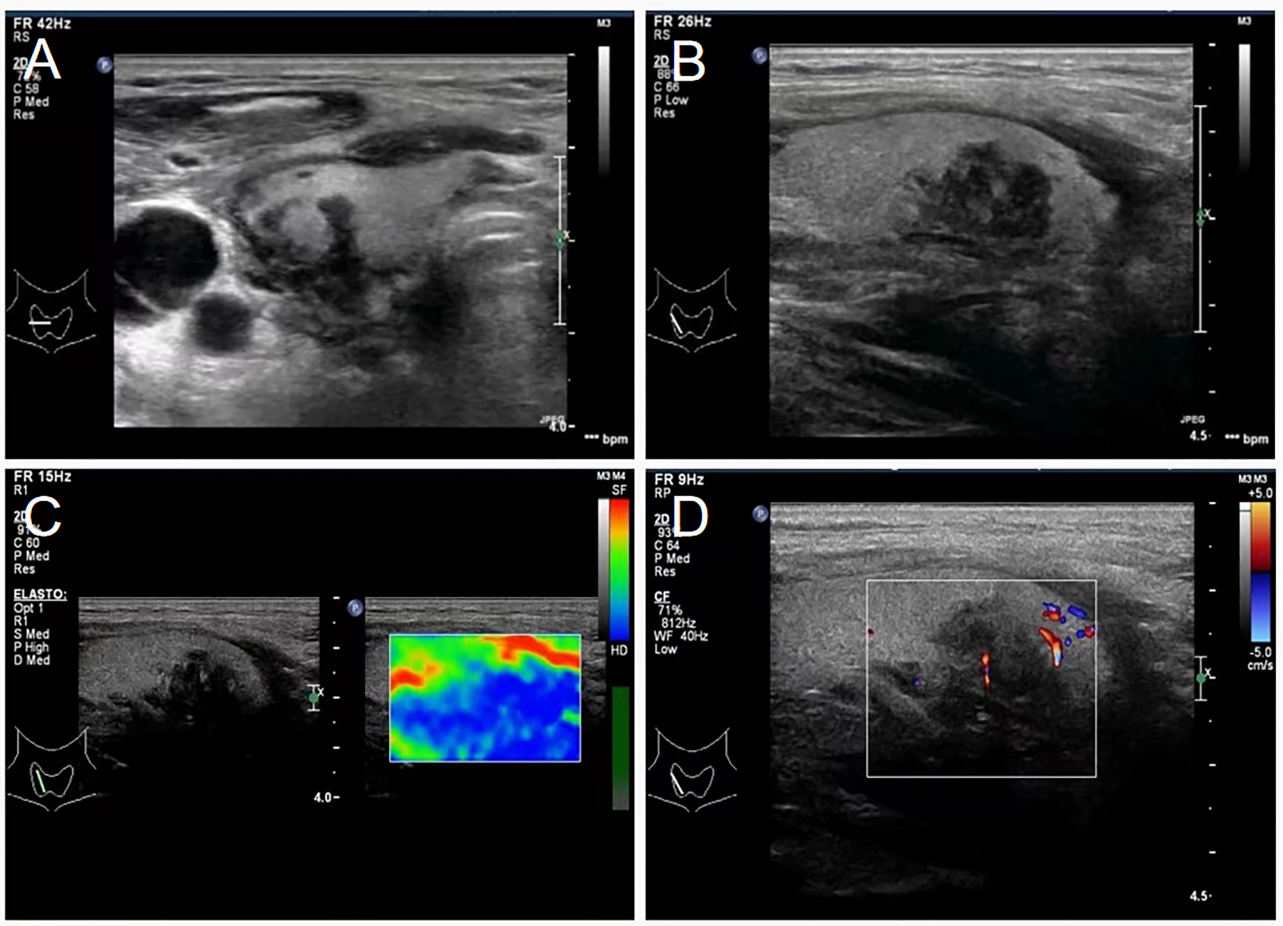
Transverse and longitudinal ultrasound showing thyroid invasion. (A) Ultrasound transects of thyroid showing a hypoechoic, irregular, and ill-defined thyroid at the right side. (B) Ultrasonography of the thyroid showing a low-echo area in the middle and lower part of the right thyroid gland, with an uneven internal echo, irregular shape, and unclear boundary. (C) Shear-wave elastography showing the hypothyroid area on the right as the essentially blue area. (D) Color Doppler ultrasonography showing minimal blood flow signal in the hypoechoic thyroid area.
